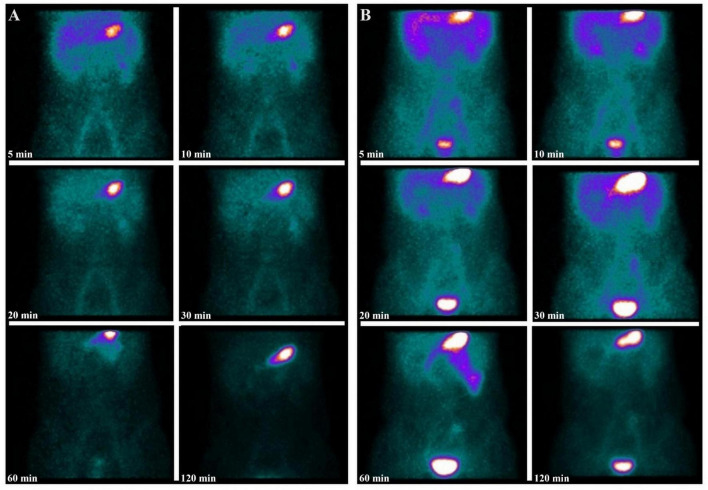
A 12-year-old boy presented with intermittent rectal bleeding and a hemoglobin level of 117 g/L. The initial serial images from [^99*m*^Tc]pertechnetate scintigraphy showed physiological radiotracer activity in the liver, stomach, and bladder, with no focal abnormal uptake suggestive of ectopic gastric mucosa in a MD (A). Given the lack of symptomatic improvement with supportive clinical management, a high suspicion of MD persisted. A repeat [^99*m*^Tc]pertechnetate scintigraphy was performed, revealing a relatively fixed area of increased radiotracer uptake in the left lower quadrant (B). The diagnosis of MD was confirmed postoperatively. Upon further inquiry, the patient’s family revealed that the patient had experienced significant rectal bleeding prior to the initial [^99*m*^Tc]pertechnetate scintigraphy, suggesting that the negative initial results might have been due to dilution of the secreted [^99*m*^Tc]pertechnetate by the bleeding.
